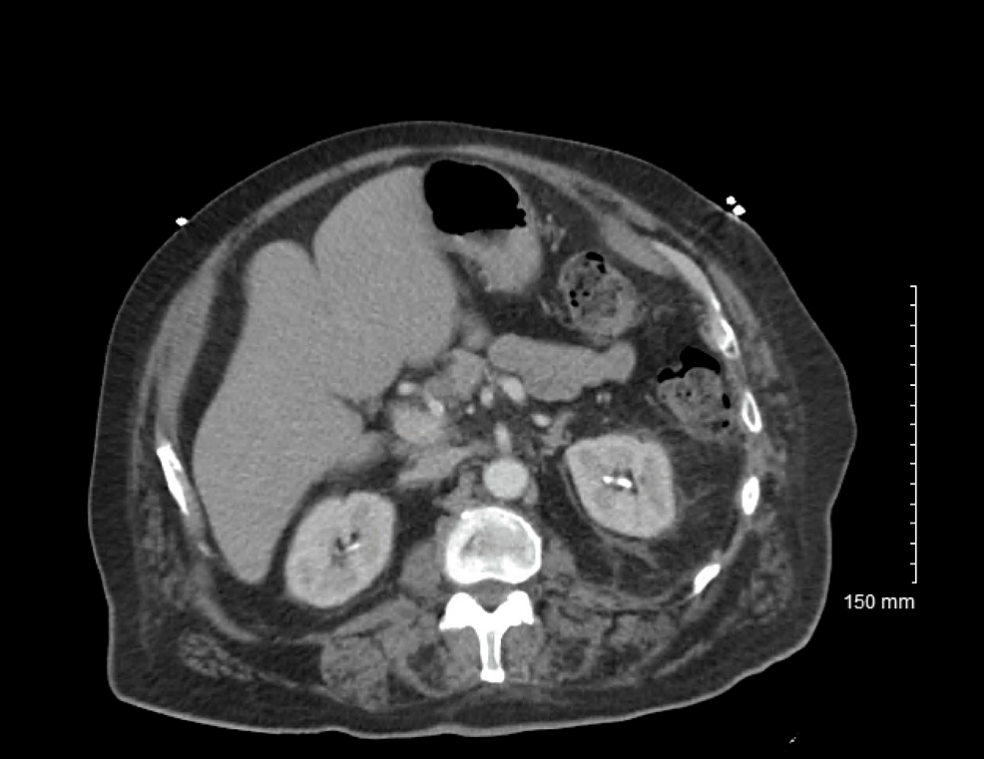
CT scan of the abdomen and pelvis with contrast.
